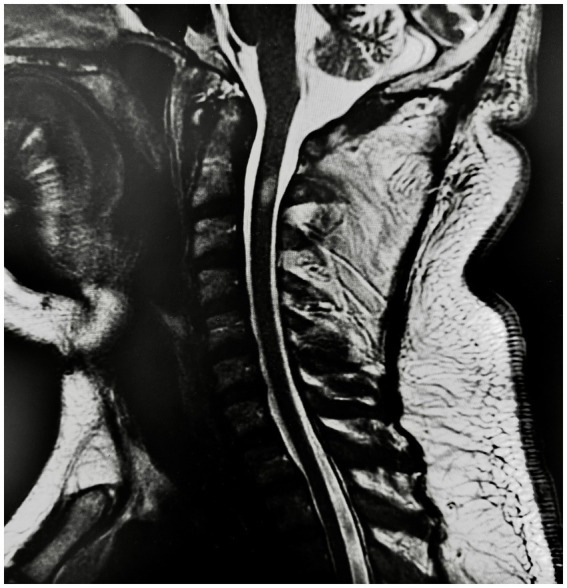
MRI of the cervical spine, T2-weighted sagittal sequence, showing a 6 mm segment of hyperintensity involving the posterolateral spinal cord at the level of C2, suggests transverse myelitis.
